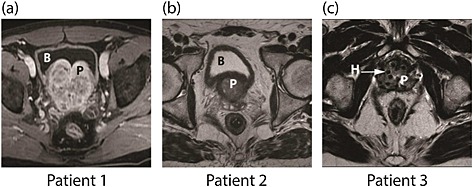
Typical MR images of the three patients selected for the study, showing patient‐specific structural features. B and P indicate the location of the bladder and prostate, respectively. (a) Patient 1: an enlarged prostate compresses the bladder; (b) Patient 2: the inferior of the bladder is tightly wrapped around the superior part of the prostate; (c) Patient 3: prostate contains holes (H) because of brachytherapy treatment.
